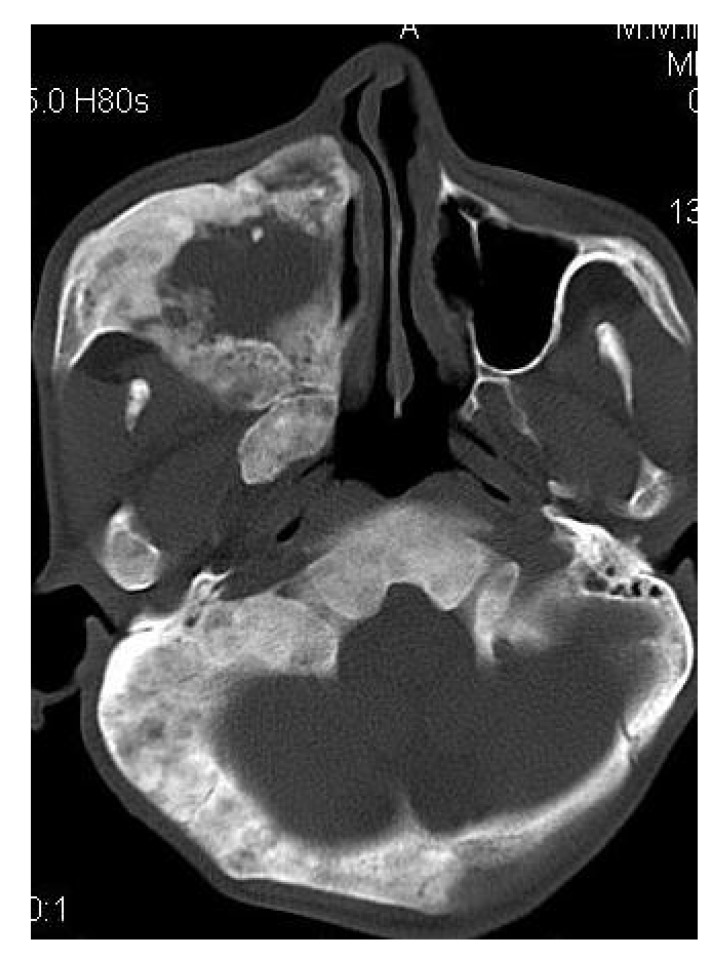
Axial CT section showing the involvement of the maxillary sinus of right side. Further the zygomatic arch, zygoma, base of the cranium, mastoid process, pterygoid plates and sphenoid of right side were also involved. There was evidence of involvement of occipital condyles of both the sides and clivus.
